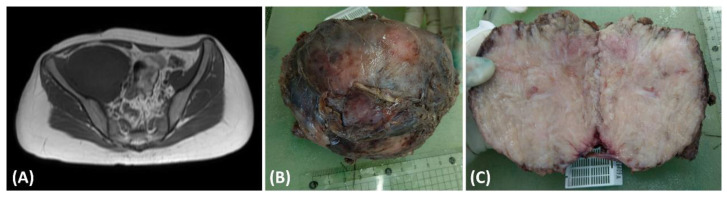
(A) The T1-weighted magnetic resonance imaging (MRI) scan exhibited a substantial intraabdominal mass originating from the retroperitoneal space, demonstrating invasion into adjacent tissues. (B) The dimensions of the excised tumor were 13.5 × 9.2 cm, characterized by fibrotic encapsulation and a firm texture. (C) Examination of the cut surface of the resected tumor disclosed a composition predominantly fleshy and fibrotic, interspersed with areas of focal central necrosis.
